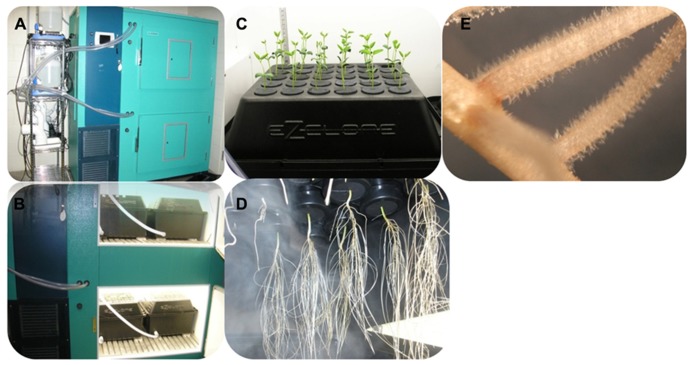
Soybean seedlings grown in the ultrasound aeroponic system; (A,B) the whole system for plant culturing; (C,D) the plants in the EZ-cloner; (E) soybean root showing a high density in root hair cells.
